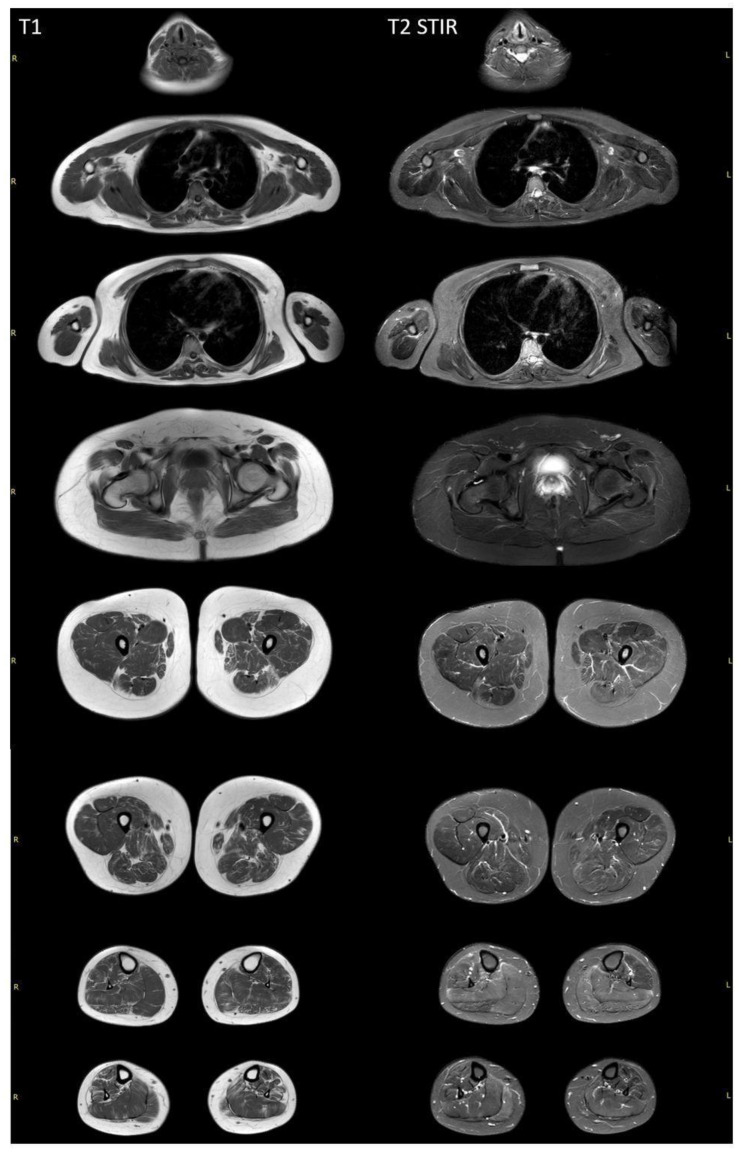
Whole-body muscle MRI of a patient with *SYNE1*-associated spinocerebellar ataxia. T1-weighted MRI revealed diffuse fatty replacement in lower limb muscles, suggesting a neurogenic pattern. In all lower limb muscles, a moth-eaten appearance is visualized, with the exception of the adductor longus and gluteus major bilaterally. Vasti muscles, as well as the medial heads of the right gastrocnemius and soleus muscles, are more preserved. The T2 STIR-weighted images demonstrate a hyperintense signal in the posterior compartment of the lower leg muscles. There are no signs of the involvement of neck, trunk, or upper limb muscles.
